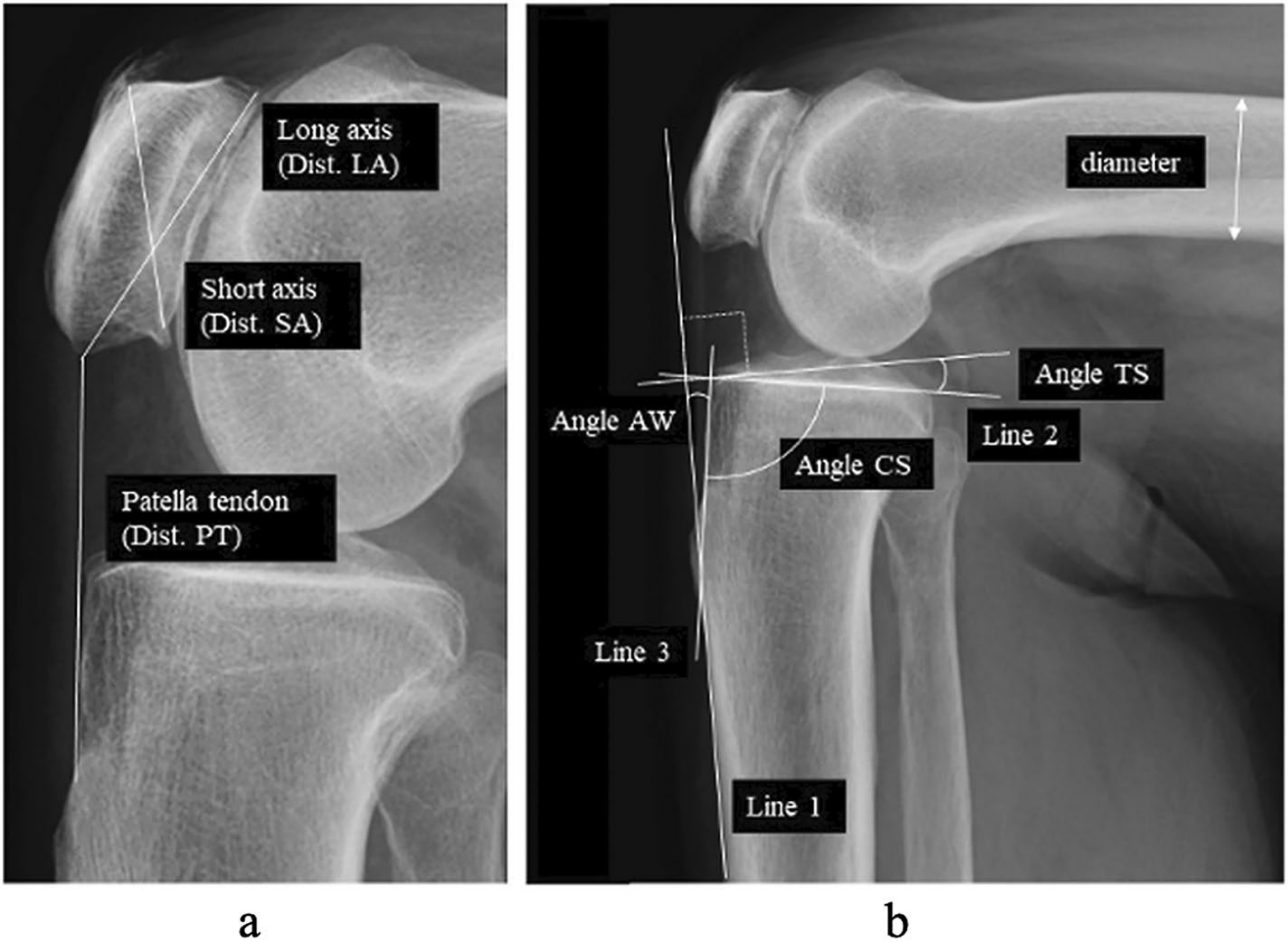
Parameters on lateral view radiograph of the knee. (a) Dist. LA: length of the long axis of the patella, Dist. SA: length of the short axis of the patella, Dist. PT: length of the patellar tendon, (b) Angle AW represents the angle of posterior rotation of the tibial condyle relative to the anterior cortex of the tibial shaft. Angle TS represents the posterior slope angle relative to the anterior cortex of the tibial shaft. Angle CS represents the degree of rhomboid transformation of the tibial condyle.
